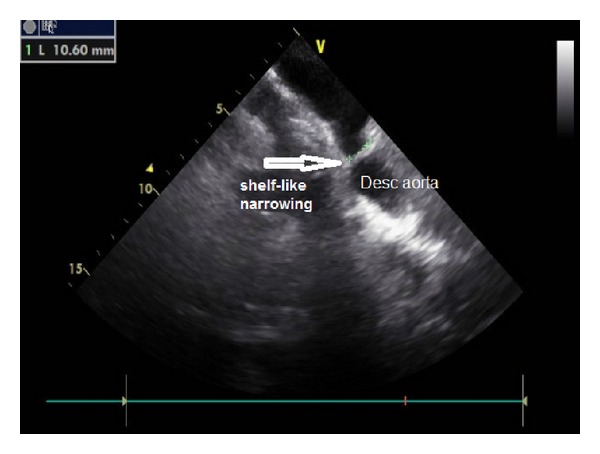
Transthoracic echocardiography showed narrowing of the descending aorta; diameter of the aortic arch was 10.60 mm.
